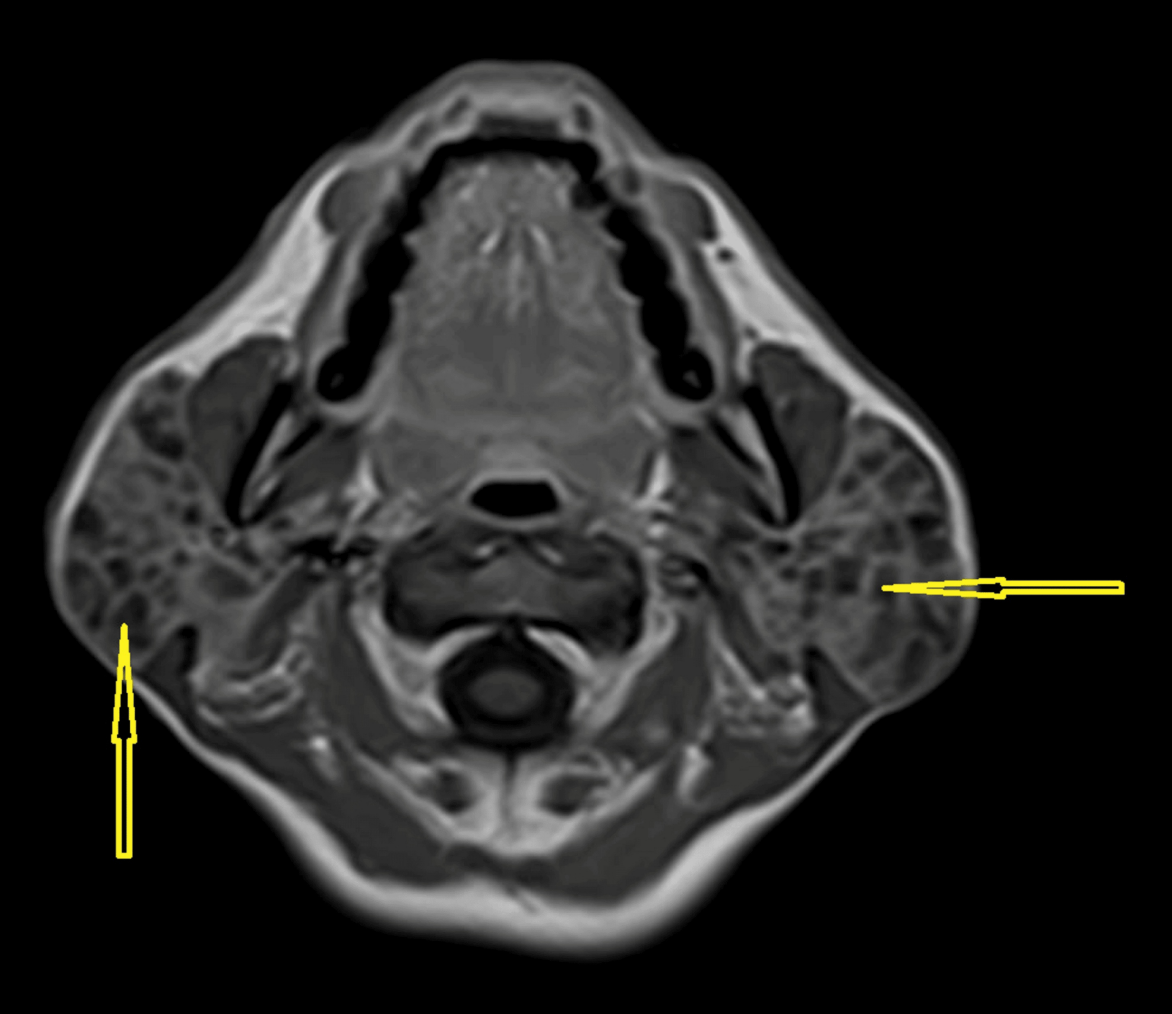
Contrast-enhanced T1-weighted axial image showing the hypointense lesions in the bilateral enlarged parotid glands
